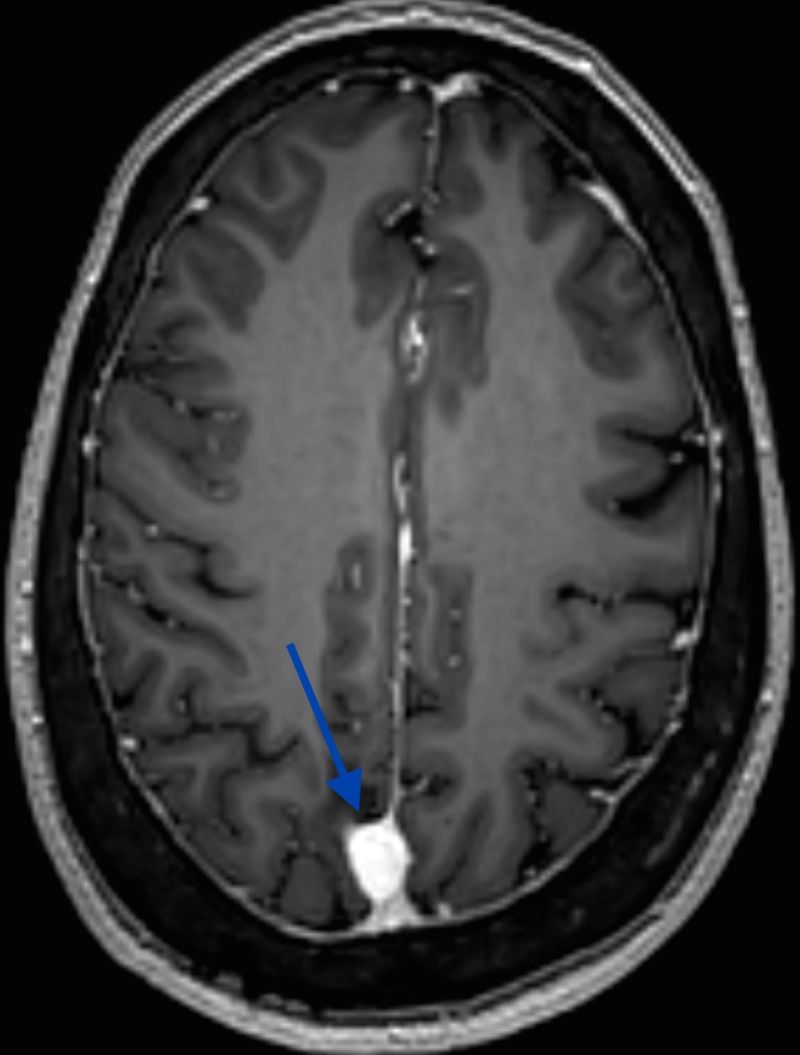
Axial T1-weighted MRI reveals the further progression of the right posterior parafalcine tumor (11x14 mm) MRI: magnetic resonance imaging
